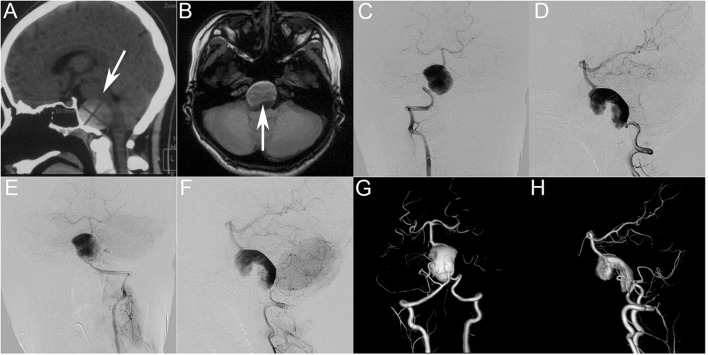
(A,B) Preoperative computed tomography (A) and magnetic resonance imaging (B) showing a giant intracranial mass effect (white arrow) in the basal ganglia region. Preoperative anteroposterior angiograms of the right vertebral artery (C,D) and left vertebral artery (E,F) showing a giant dissecting aneurysm in the vertebrobasilar artery. (G,H) Three-dimensional reconstruction showing that the dissecting aneurysm straddles bilateral vertebral arteries and that the anterior inferior cerebellar artery arises from the aneurysm.
